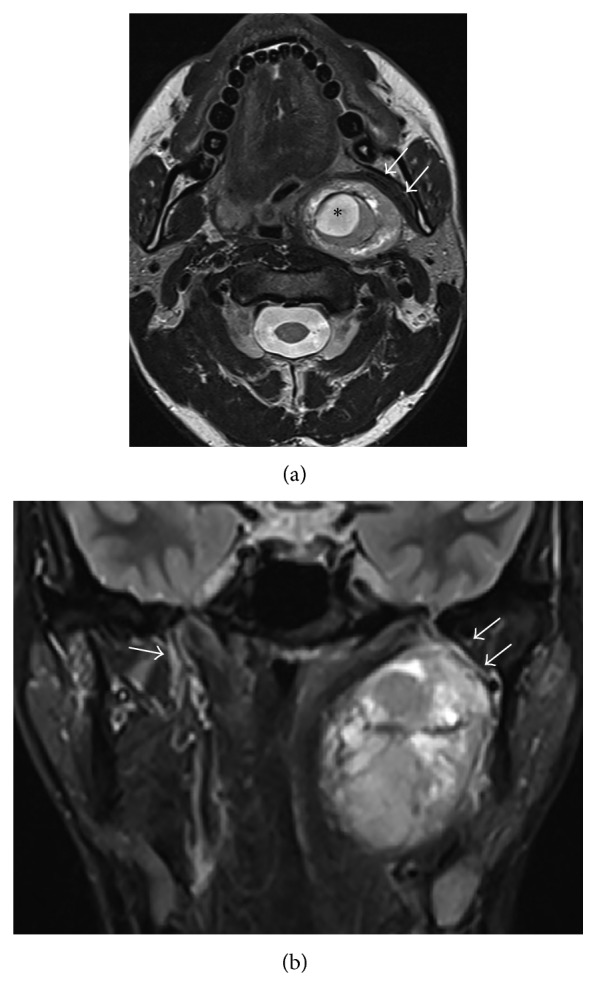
(a) Magnetic resonance imaging, axial projection. A large mass with cystic degeneration (asterisk) dislocating the root of the tongue is seen in the left parapharyngeal space. Stretched pterygoid muscles are shown with arrows. (b) Coronal projection shows the limits of the tumor (upper: the skull base; lower: the level of the submandibular salivary gland). Double arrow shows the laterally dislocated third branch of the trigeminal nerve on the left side. Note the normal proximal segment of the V3 on the right side (thin arrow).
